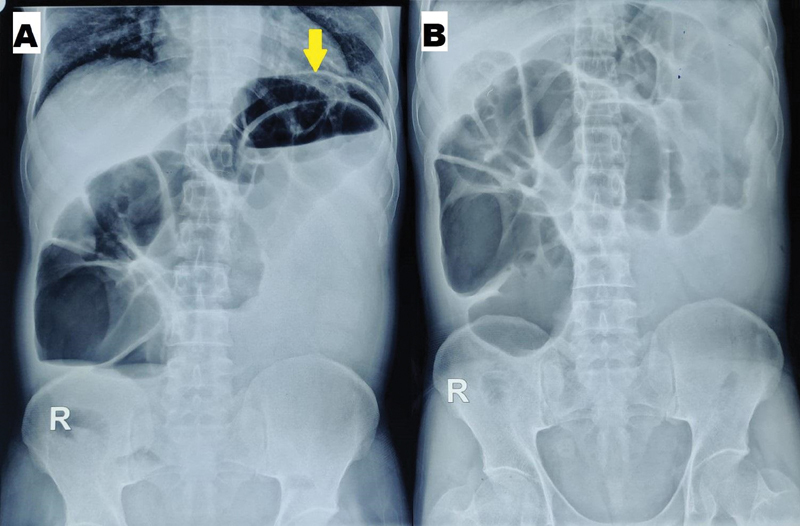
Abdominal radiograph showing (
